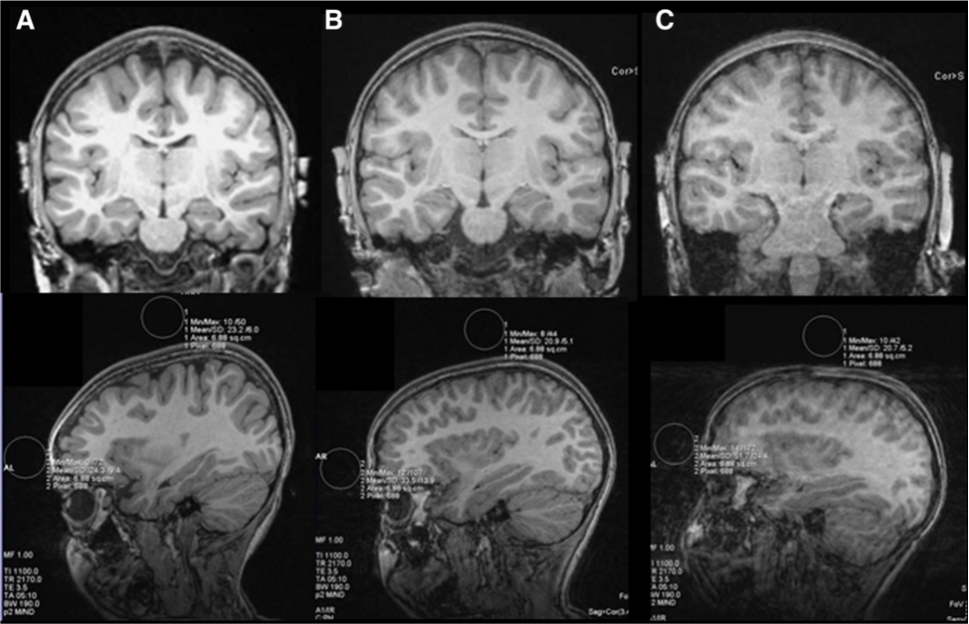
Examples of T1-weighted images with varying degrees of motion. Coronal slices of the scans (*top*) and sagittal slices depicting the motion QA assessment for a a scan from with minimal motion (QA ratio = 1.05; participant 2 in Tables 1 and 2), b a scan with moderate motion (QA ratio = 1.48; participant 4 in Tables 1 and 2), and c an unsuccessful MPRAGE attempt for the same participant depicted in b demonstrating an unacceptable level of motion (QA ratio = 2.5)
